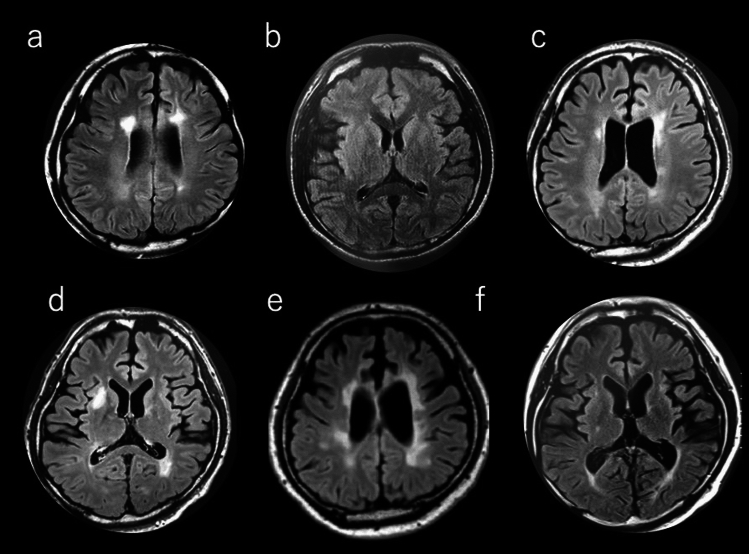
Representative case of disseminated encephalomyelitis-like lesions. Brain magnetic resonance (MR) images from case 23, exhibiting atypical features in lesion location, morphology, and gadolinium (Gd) enhancement pattern. Initial MRI at onset (a,b) shows multiple edematous lesions involving the periventricular white matter (b) with irregular, jagged Gd enhancement. Three years later (c, d), new disseminated lesions appeared in the bilateral centrum semiovale, right basal ganglia (d), and pons. At 10-year follow-up (e, f), brain atrophy became evident, and confluent lesions were observed in the bilateral centrum semiovale, periventricular white matter (e), and middle cerebellar peduncles. Although some lesions were located in the periventricular white matter, this case was classified as disseminated encephalomyelitis-like lesions because the lesions showed atypical morphology for conventional MS, including relatively large and edematous lesions at initial presentation (a, b), involvement of the right basal ganglia during follow-up (d), and regression or disappearance of some pre-existing lesions over time (e)
